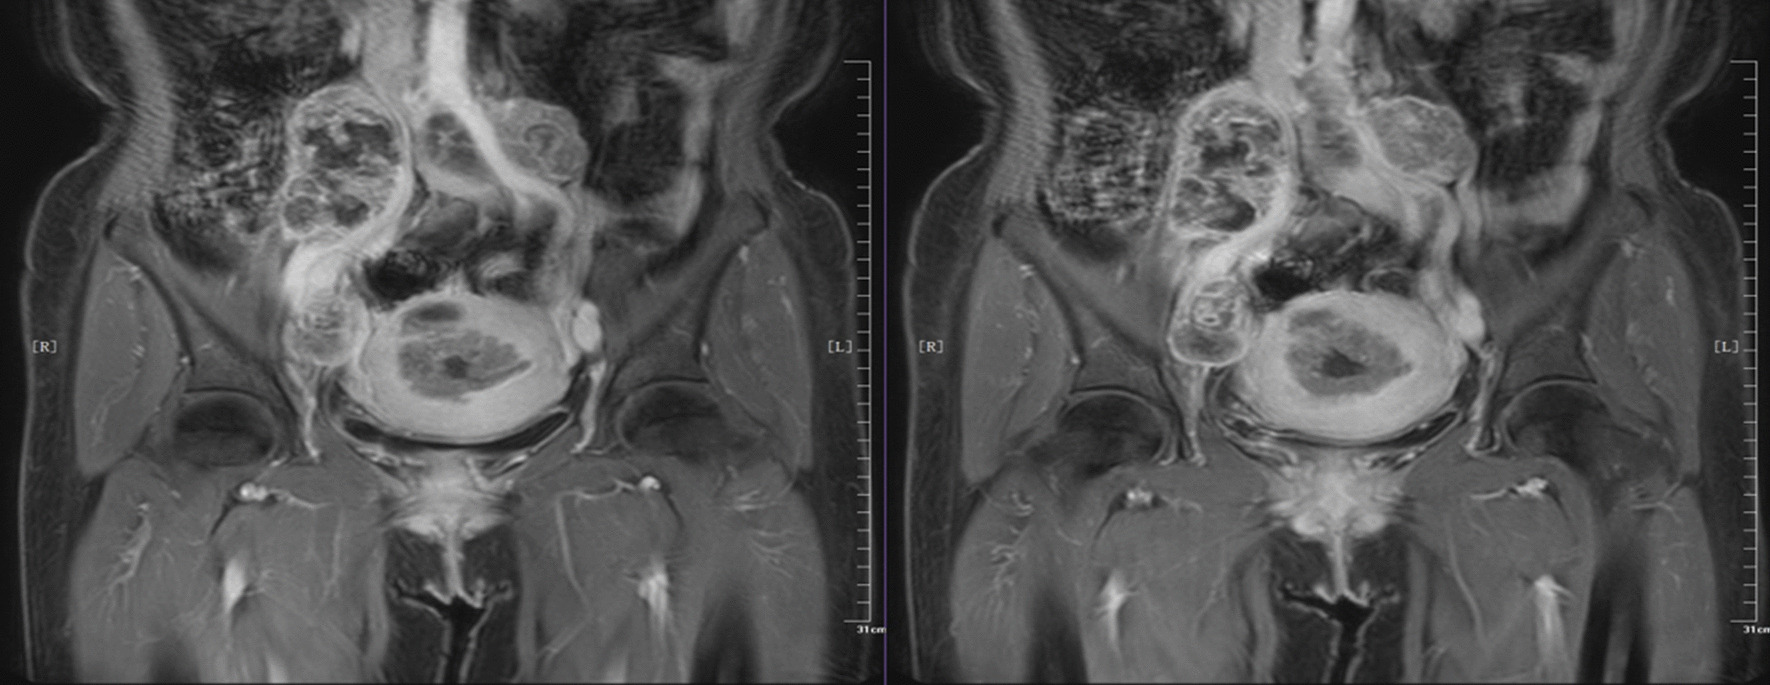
Magnetic resonance imaging (MRI) of the endometrial. Multiple lymph nodes were enlarged retroperitoneally and adjacent to the right iliac vessels, which were adherent and wrapped around the vessels
